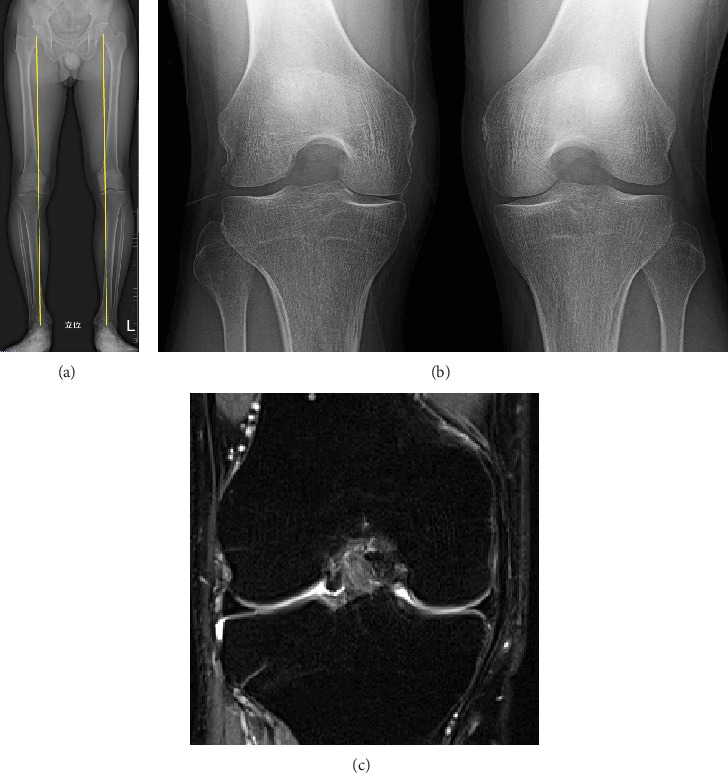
Postoperative images taken 2 years after revision meniscal repair. (a) The whole leg standing radiograph shows the same extent of degree of varus alignment in the bilateral leg. (b) Postoperative plain radiograph using the Rosenberg view shows no further progression of osteoarthritic changes in the right knee compared to the preoperative images. (c) MRI demonstrated continuity between the MM and the joint capsule without joint fluid infiltration at the interface.
